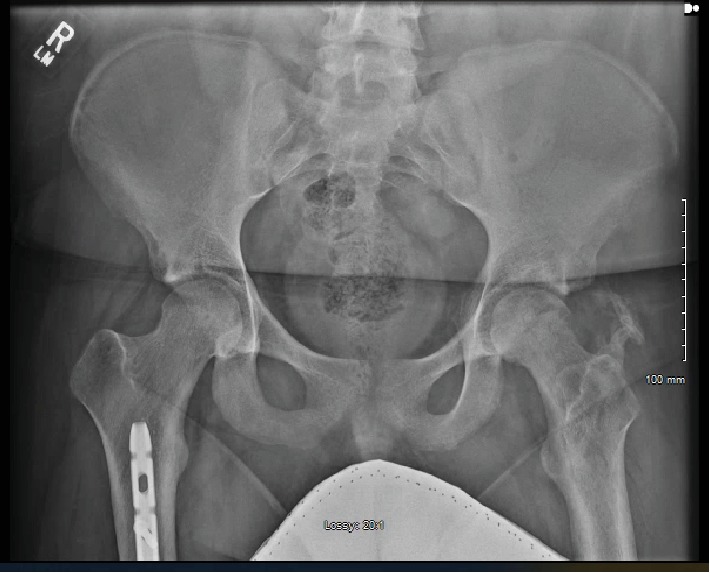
Follow-up AP pelvis radiograph at 4.5 months postinjury demonstrating further heterotopic ossification and some irregularity of the femoral head concerning for osteonecrosis.
